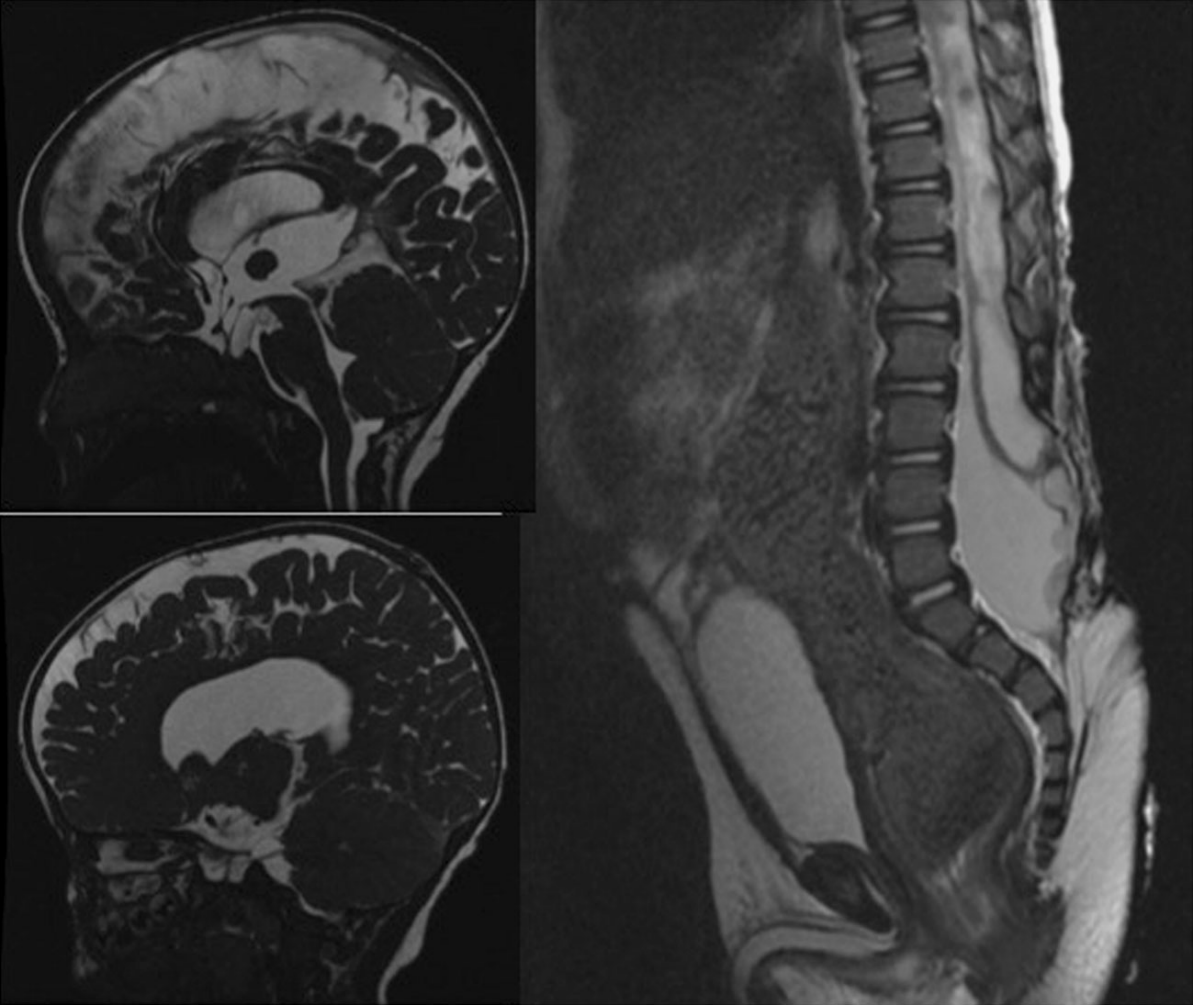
T2-WI MRI of the lumbosacral and skull in a male patient that is 8 months—age that underwent a fetal surgery at 25 weeks of pregnancy showing an absence of intracranial hypertension, patency of the aqueduct, cerebellum in the normal position, and subdural cerebrospinal fluid effusion as a benign hydrocephalus. In the sagittal view is represented a large spinal dysraphism from L2 to S4, showing syringomyelia, tethered cord, and epidermoid tumor
